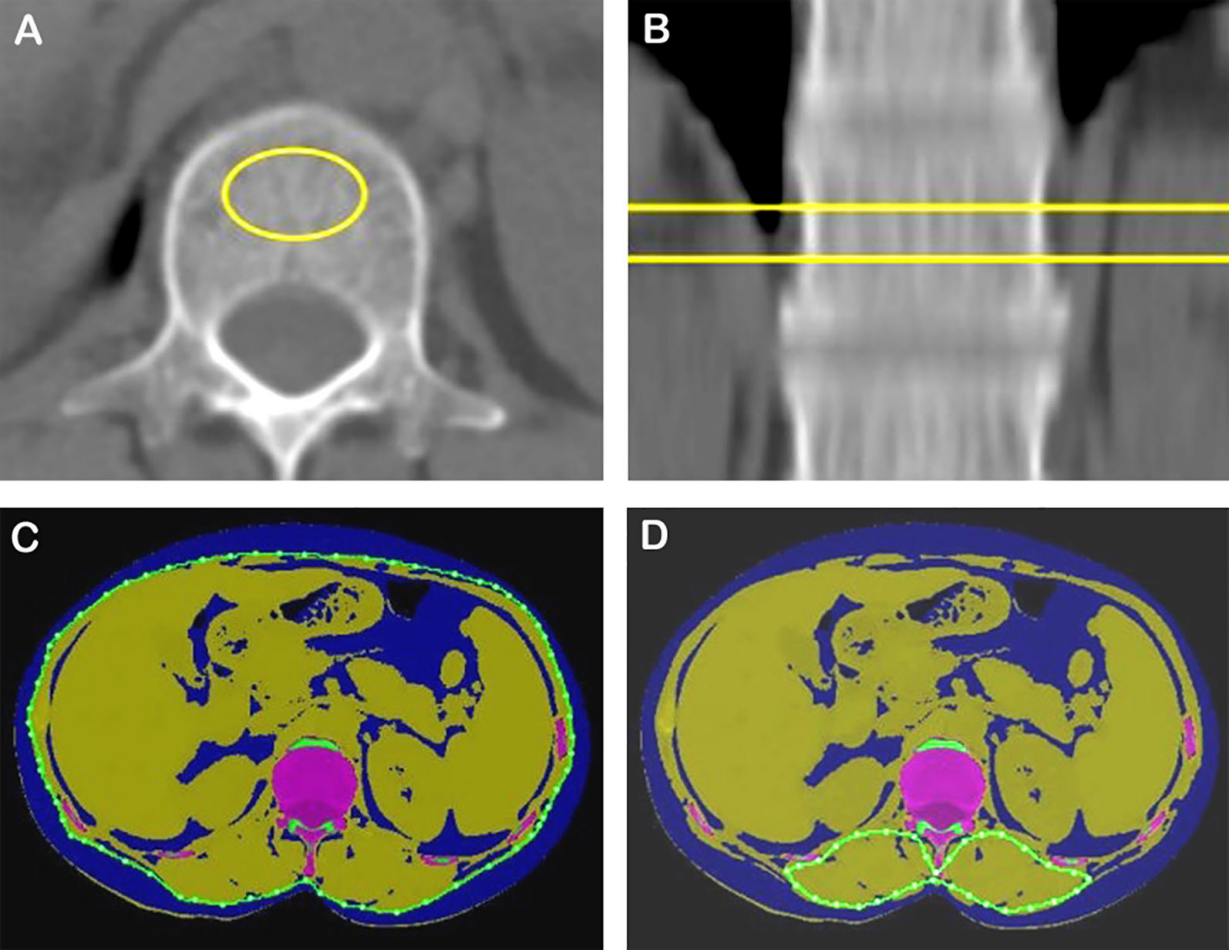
vBMD, skeletal muscle, and adipose tissue areas quantified using a single CT section at the first lumbar level. (A, B) Measurement of vBMD. (C) Assessment of SAT and VAT (blue). (D) Measurement of posterior vertebral muscle. vBMD, volume bone mineral density.
